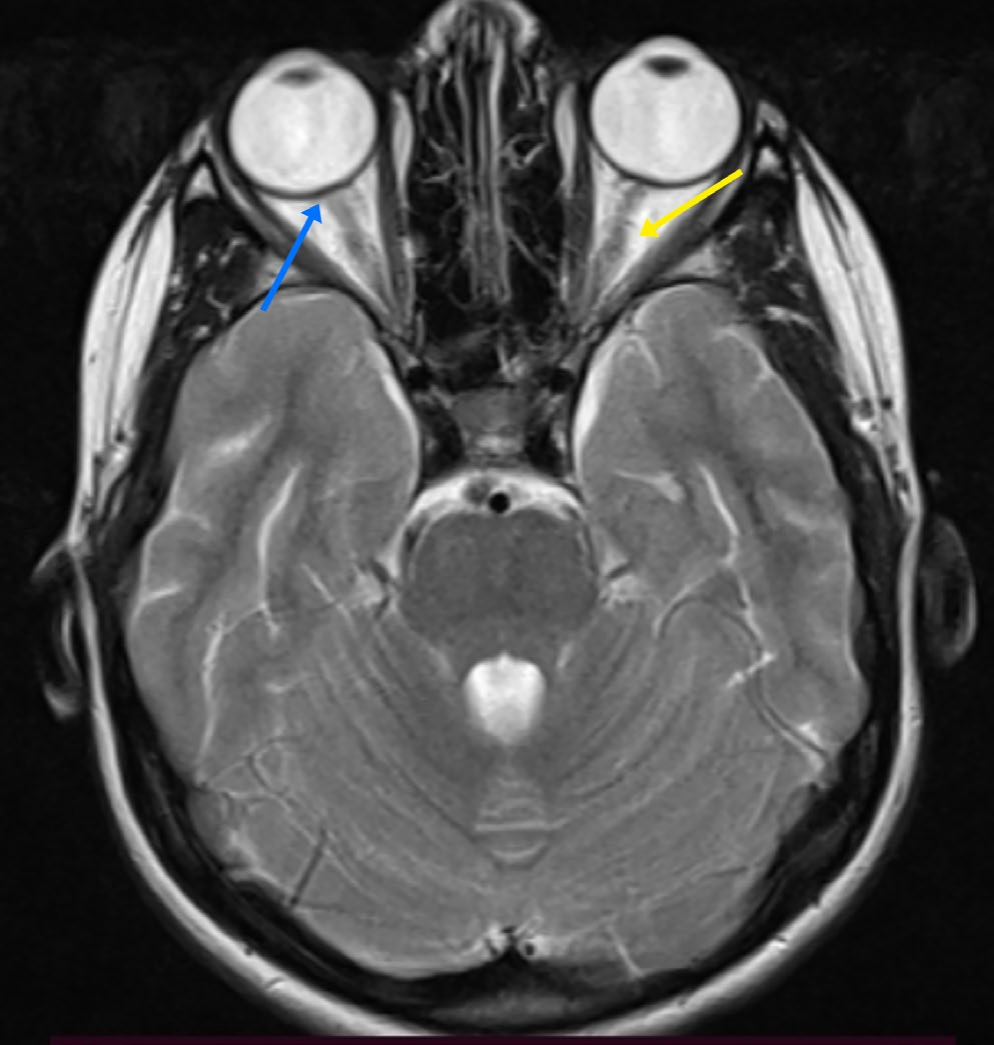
Brain magnetic resonance imaging showing mild optic nerve tortuosity (yellow arrow) and posterior globe flattening (blue arrow)
